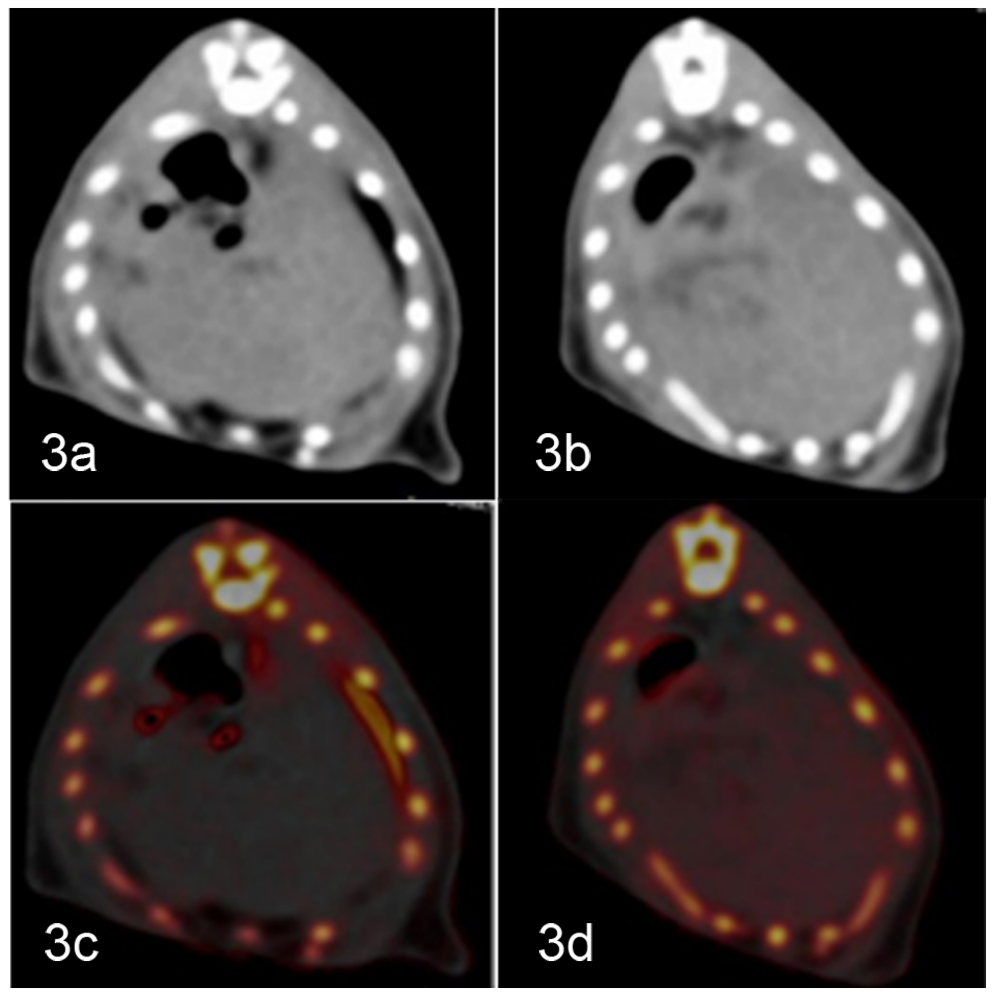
Examples showing mild and severe iron deposition; steatosis also coexisted in the liver. a–b) In the general view image, the degree of iron or steatosis in the liver could barely be determined from the gray-scale or CT value. c–d) On the VIC image, the existence and degree of iron are indicated in red colored dots and the iron enhancement value; the existence and degree of steatosis can be measured by the VNC value. For heavier iron deposition, more intense red colored dots and higher iron enhancement values were observed. More severe steatosis resulted in a smaller VNC value.
